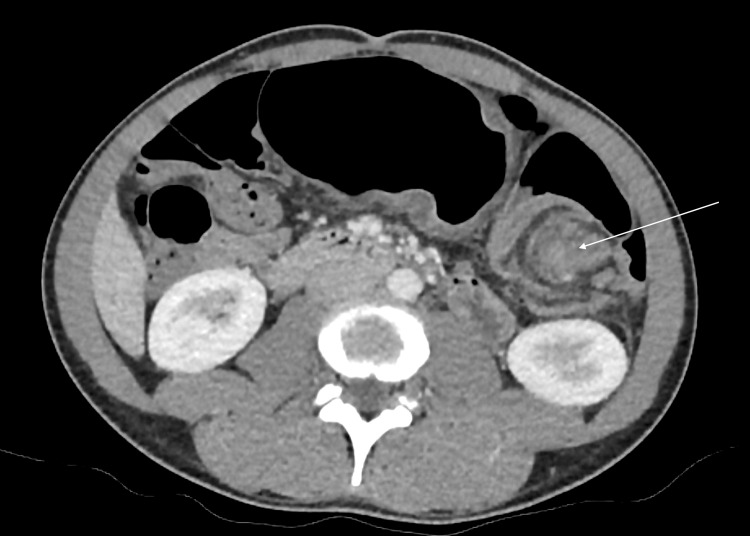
Axial CT view of the abdomen. Dilated large bowel loops with a *whirl sign* of the mesentery (arrow) are seen in the left upper quadrant of the abdomen.
